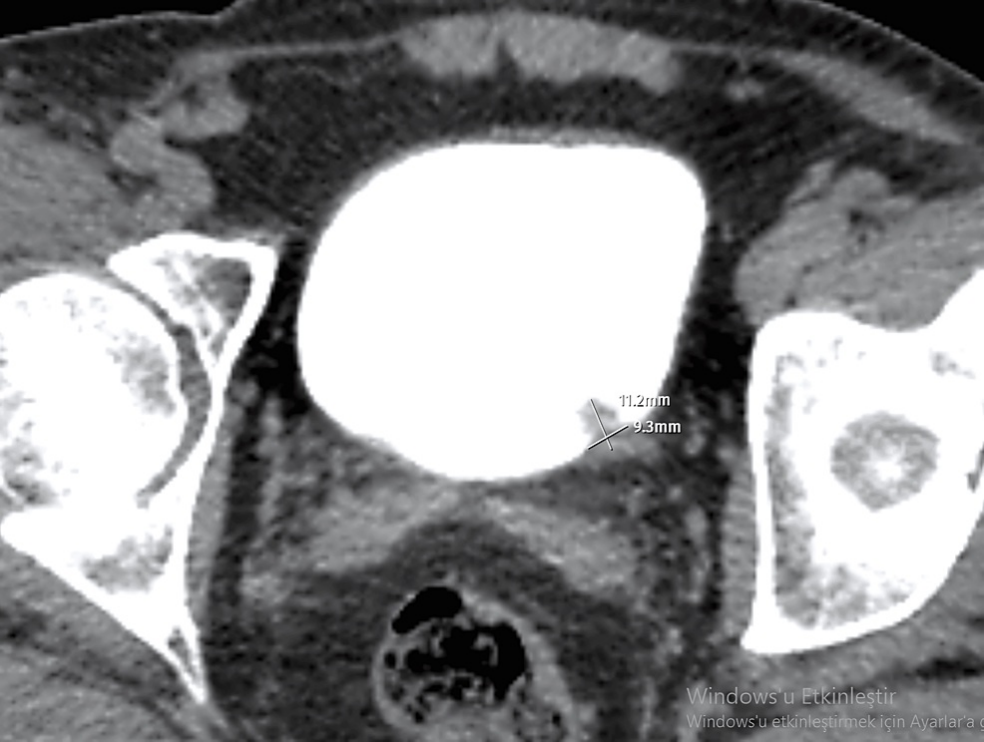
Axial CT section of the bladder tumor in a 63-year-old male patient.
